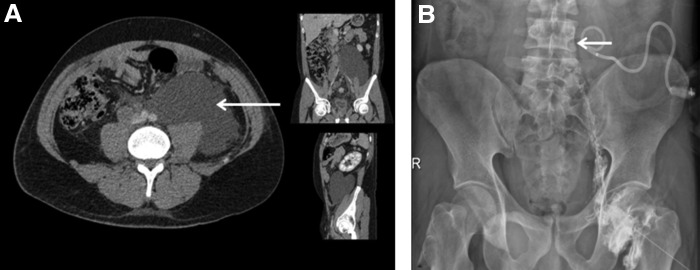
(A) Abdominal CT scan axial, coronal, and sagittal showing retroperitoneal chyle leak (*arrow*). (B) Lymphangiography shows the extravasated iodixanol (*arrow*) at fourth lumbar spine level within retroperitoneum.
